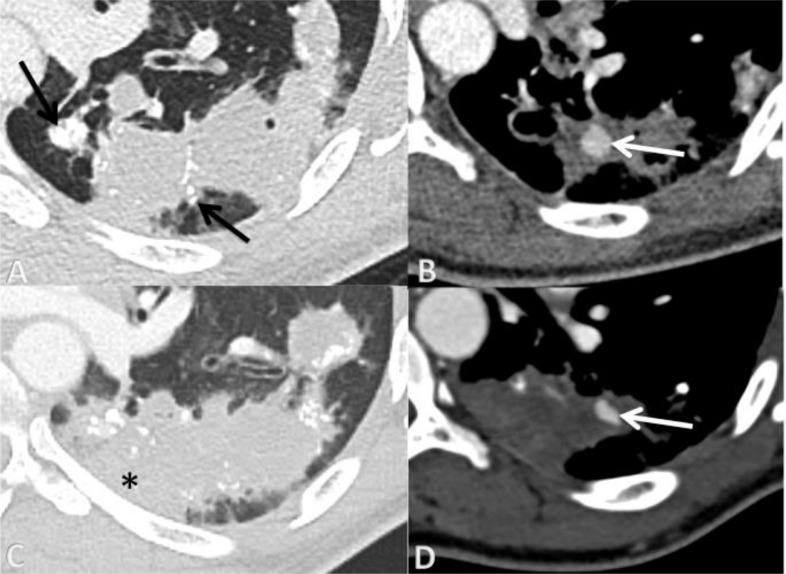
(A) Chest CT scan performed on the patient's emergency arrival in parenchymal section shows an alveolar condensation of the left lower lobe, with some nodular calcifications, suggestive of tuberculosis sequelae. (B) Axial chest CT angiogram shows a PAP of the left lower lobe measured at 9 × 9 mm, compatible with a PAP (whit arrow). (C) Chest CT scan in parenchymal section performed 7 days after arrival showed increased alveolar condensation of the left lower lobe (*). (D) Chest CT angiogram confirmed the persistence of a 4 × 9 mm pseudoaneurysm.
